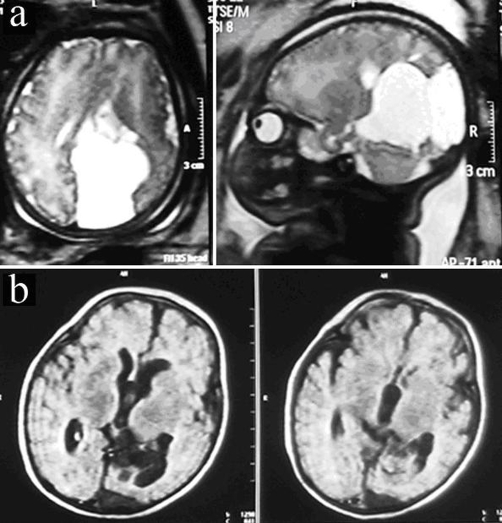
(A) A fetal MRI was showed multiseptal and subcortical cystic lesions on the right occipital lobe that originated from right lateral ventricle. (B) Annual MRI shown no recurrence of the CPC or septal membrane at post-operative 3 years.
